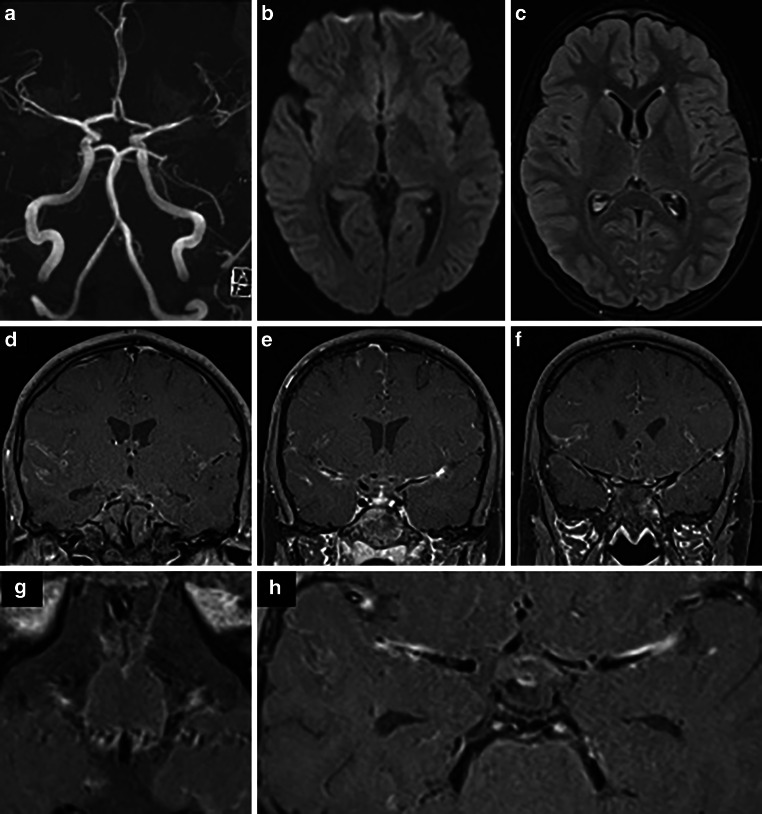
MRI of a 13-year-old boy obtained 1 day after the clinical onset of cerebral vasculitis due to LNB. MIP images of TOF angiography (a) demonstrated better arterial flow in the left MCA branches. DWI (b = 1000 s/mm2) (axial view; b) and FLAIR images (axial view; c) showed a decrease in ischemic changes within the left insular cortex. Fat-saturated contrast-enhanced T1-weighted images (coronal views: d, e, f; axial views: g, h) revealed diffuse leptomeningeal enhancement and arterial wall thickening with contrast enhancement in the both MCA and basilar artery
